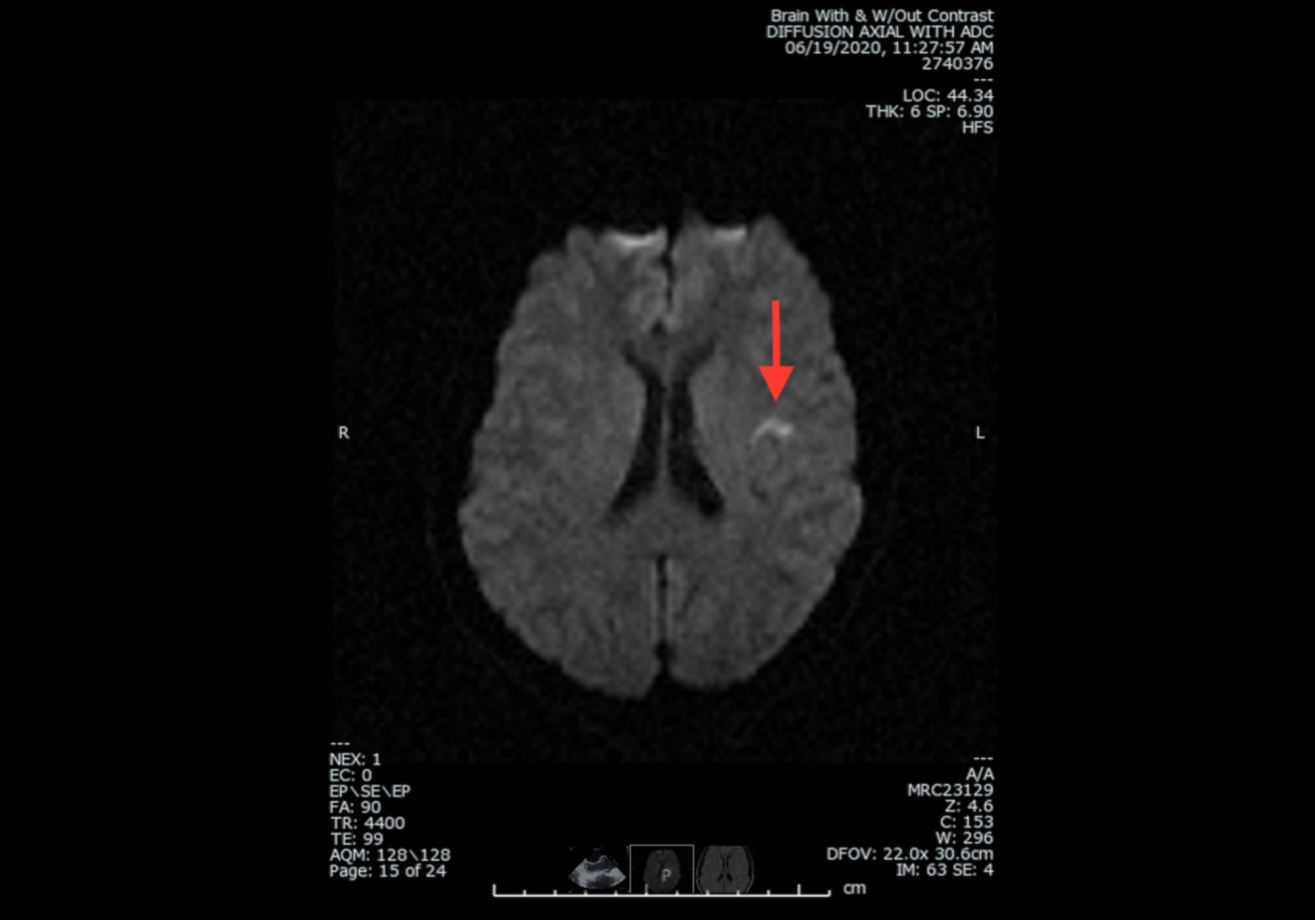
MRI head with and without contrast depicting a recent cortical infarct in left frontal region.
